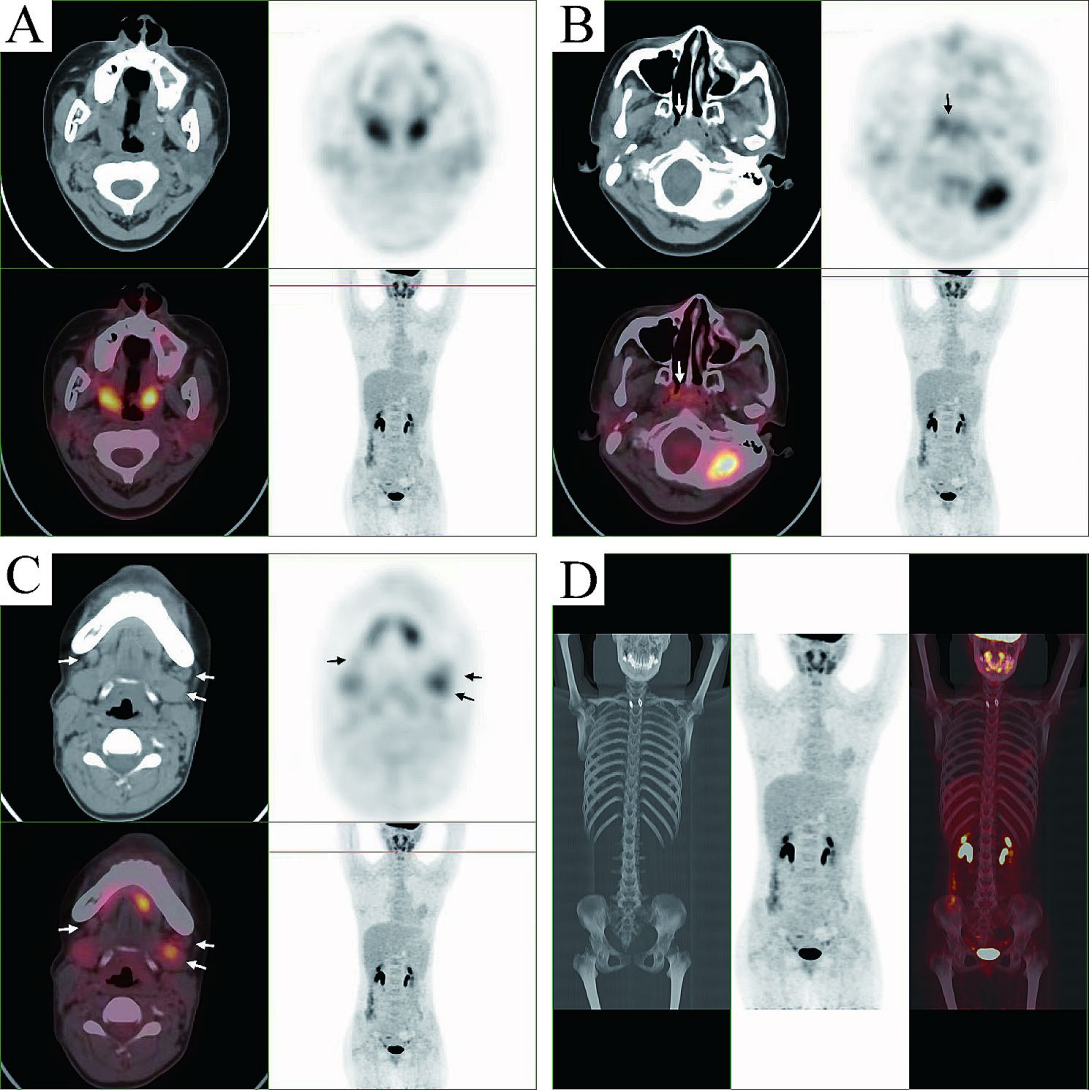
Positron emission tomography/computed tomography (PET/CT) indicated infiltration to the throat (A), maxillary (B), and submandibular glands (C). (D) No obvious distant metastasis was detected
